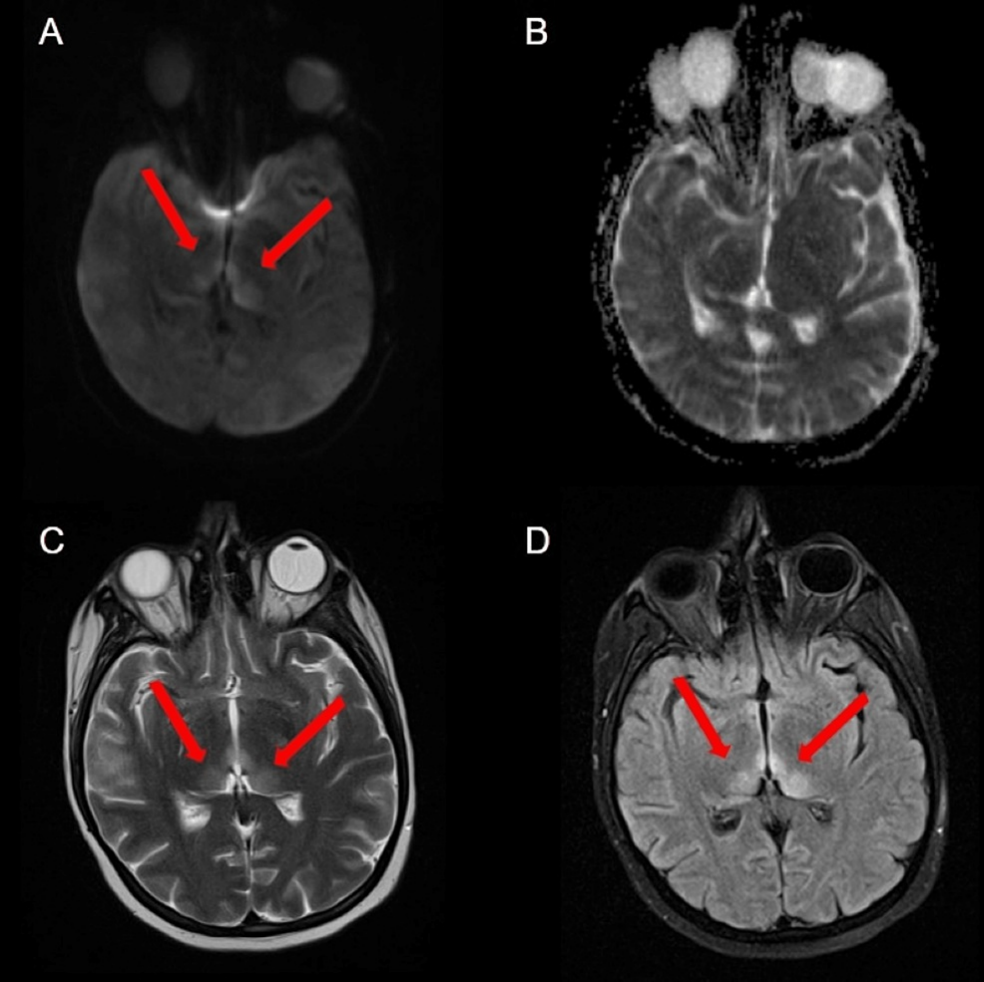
MRI brain without contrast MRI brain without contrast showing abnormal symmetric hyperintensities within the bilateral thalami (red arrows) displayed on axial DWI (A), axial T2 (C), and axial FLAIR (D). There is no increased signal in the bilateral thalami on ADC sequence (B), indicating diffusion restriction in the bilateral thalami. DWI, diffusion-weighted imaging; FLAIR, fluid-attenuated inversion recovery; ADC, apparent diffusion coefficient.
